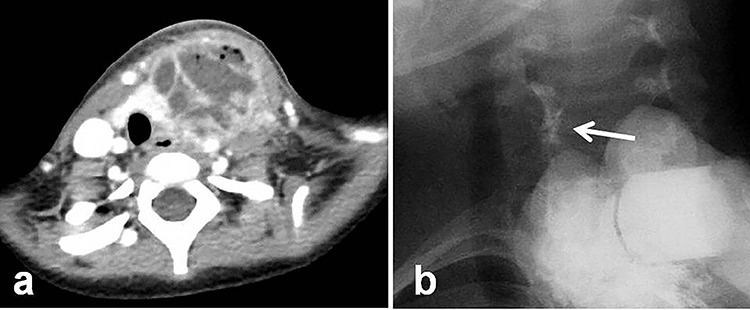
Radiologic examinations. (a) Enhanced computed tomography showing a huge abscess. (b) At the second hospitalization, direct fistulography from the wound showed an obvious fistula from the abscess cavity to the left pyriform sinus (white arrow: fistula).
